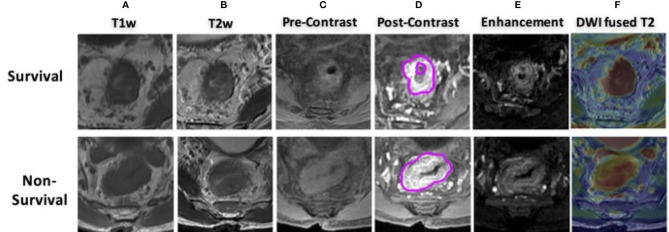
MR images of two male patients, both at 60 years old with mid-rectum cancer at stage cT3N+M0, pretreatment (A) T1-weighted image, (B) T2-weighted image, (C) precontrast image, (D) 60 s after contrast injection image, (E) subtraction image showing difference between panels (D, C, F) the derived apparent ADC map fused to a T2-weighted image. The first row shows a case as 5-year survival patient, and the bottom shows a non-survival case.
